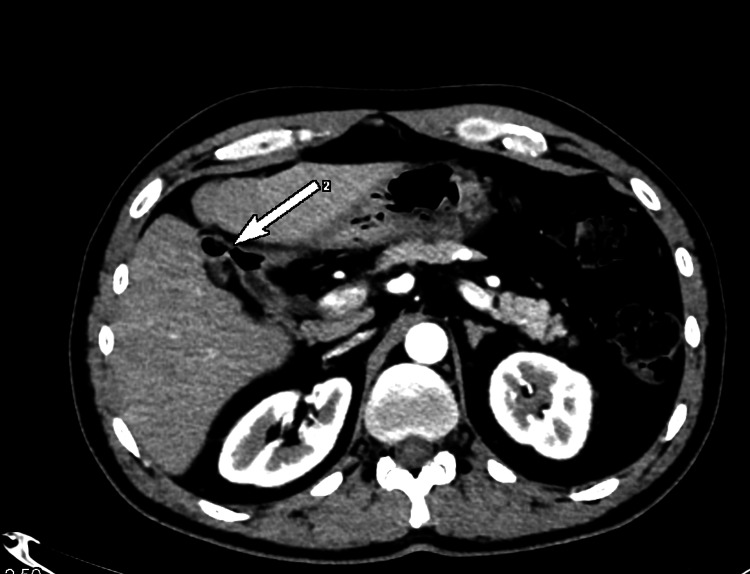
Computed tomography, axial view; a fistula between the corpus of the gallbladder and the bulb of the duodenum (white arrow).
